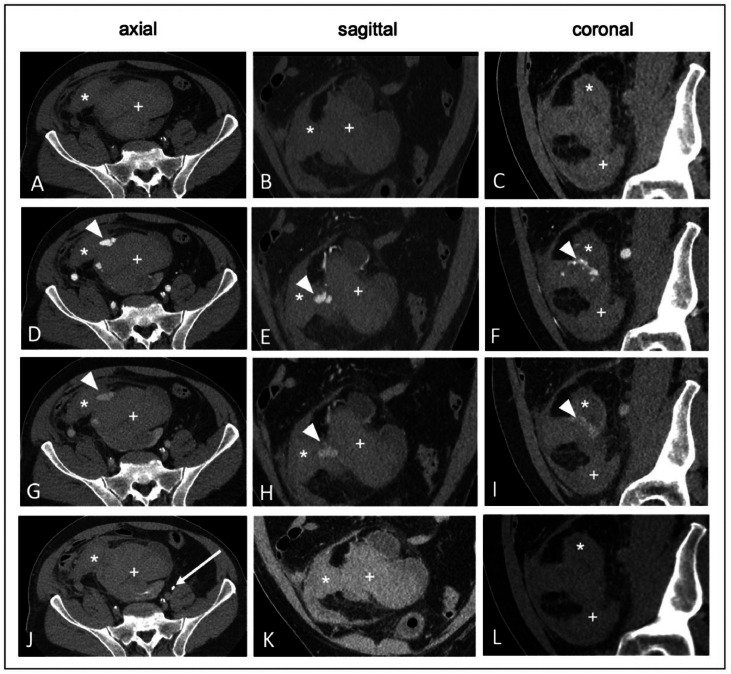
CT images of the pelvis, including one native (noncontrast) phase (A-C) and three contrast-enhanced phases: arterial (D-F), venous (G-I), and urographic (J-L). Key findings are highlighted: ► indicates findings suspicious for vascular pathology (eg, contrast extravasation or aneurysm); * denotes hematoma; + represents the pelvic kidney/hematoma complex; and an arrow in (J) points to the contrast enhancement of the left ureter.
